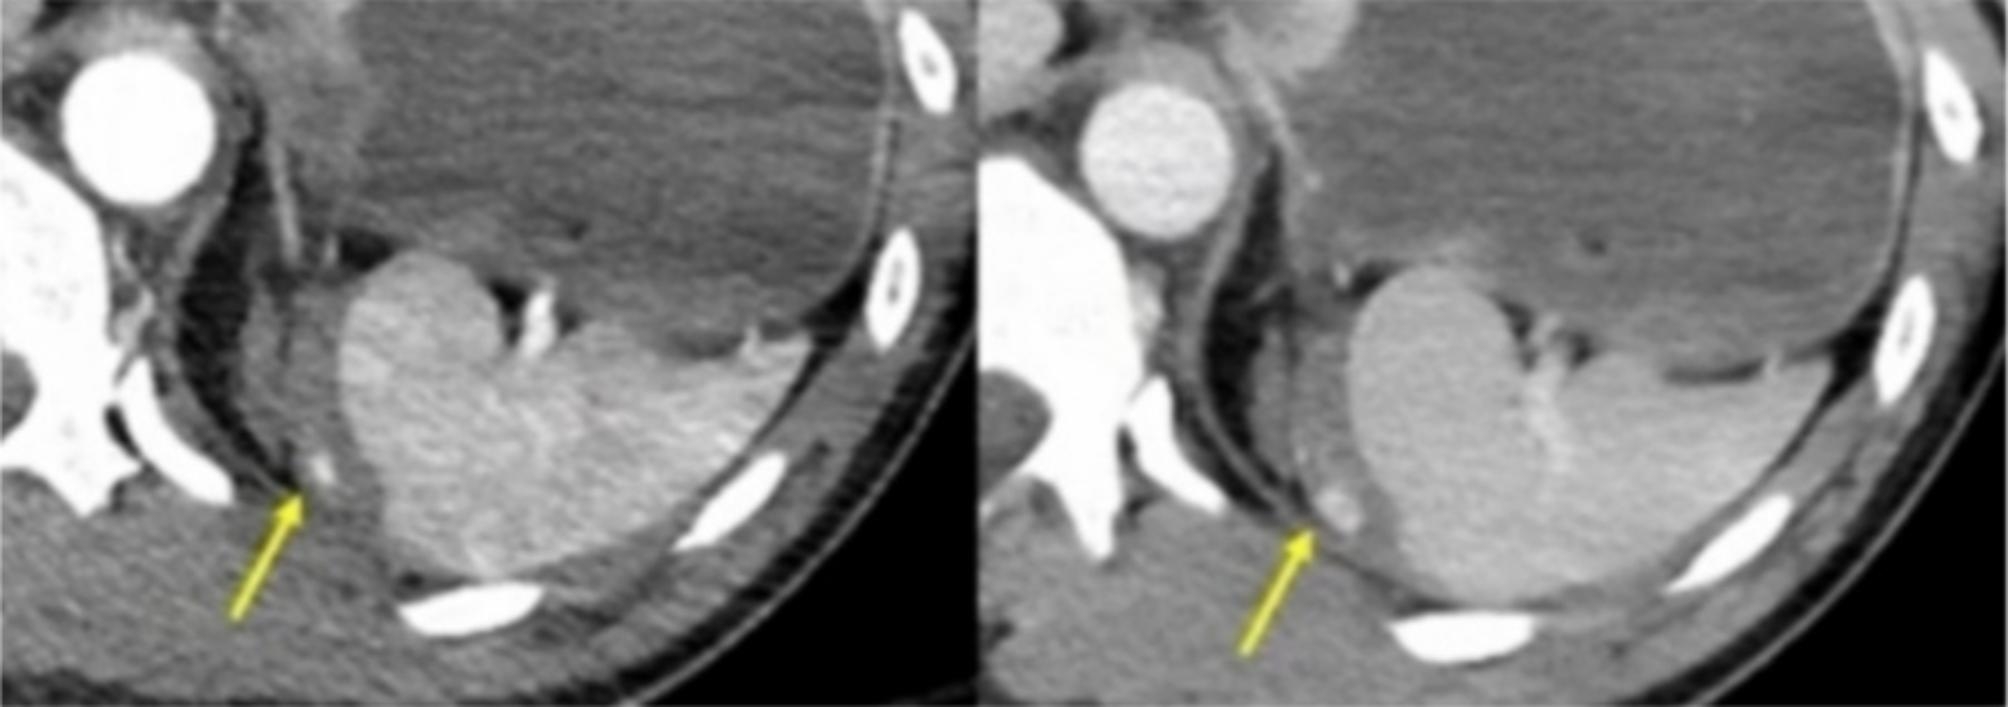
CT for partial splenic tear
